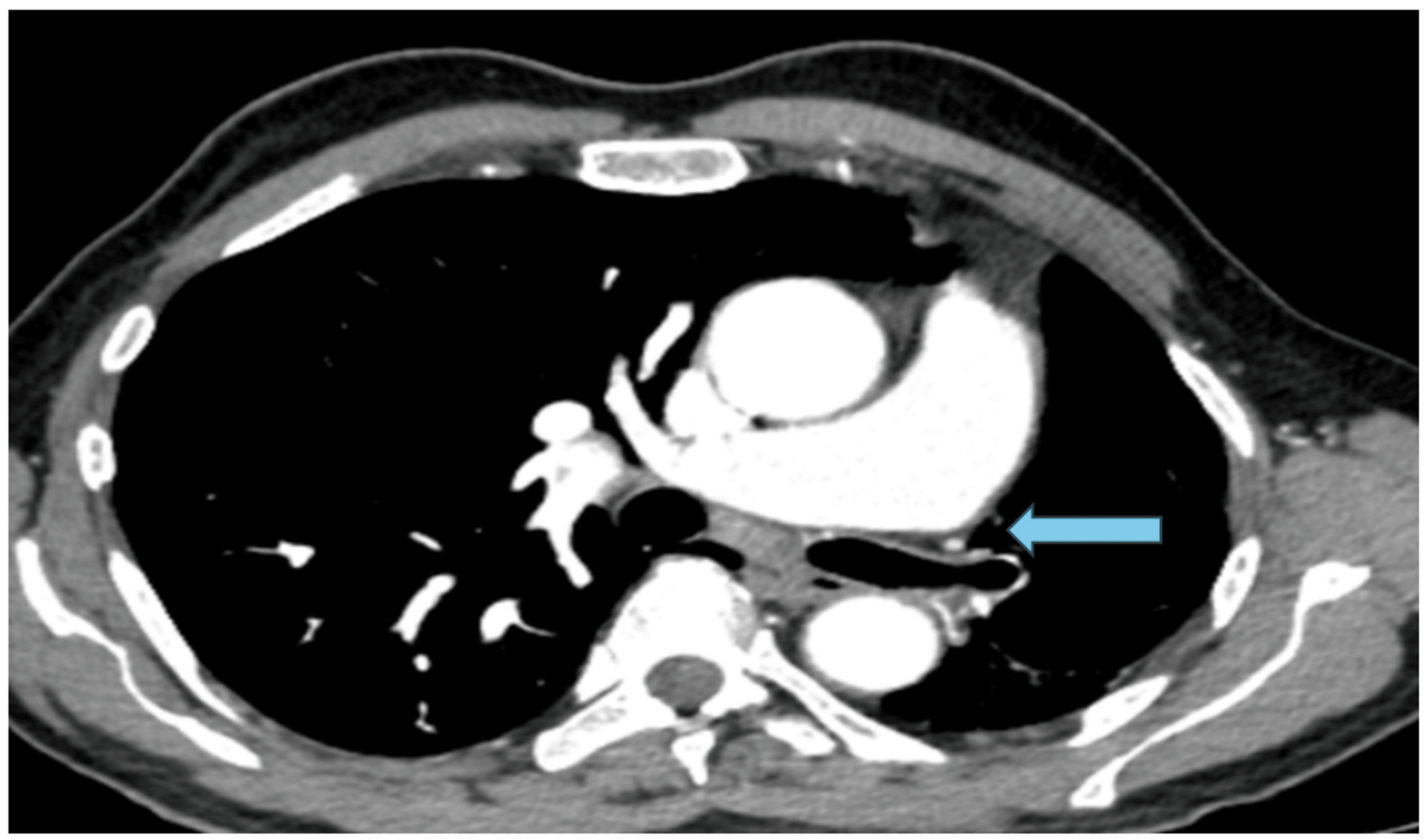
Axial CECT chest (mediastinal window) shows reduced caliber of the left pulmonary artery which was hypoplastic. CECT: Contrast-enhanced computed tomography
